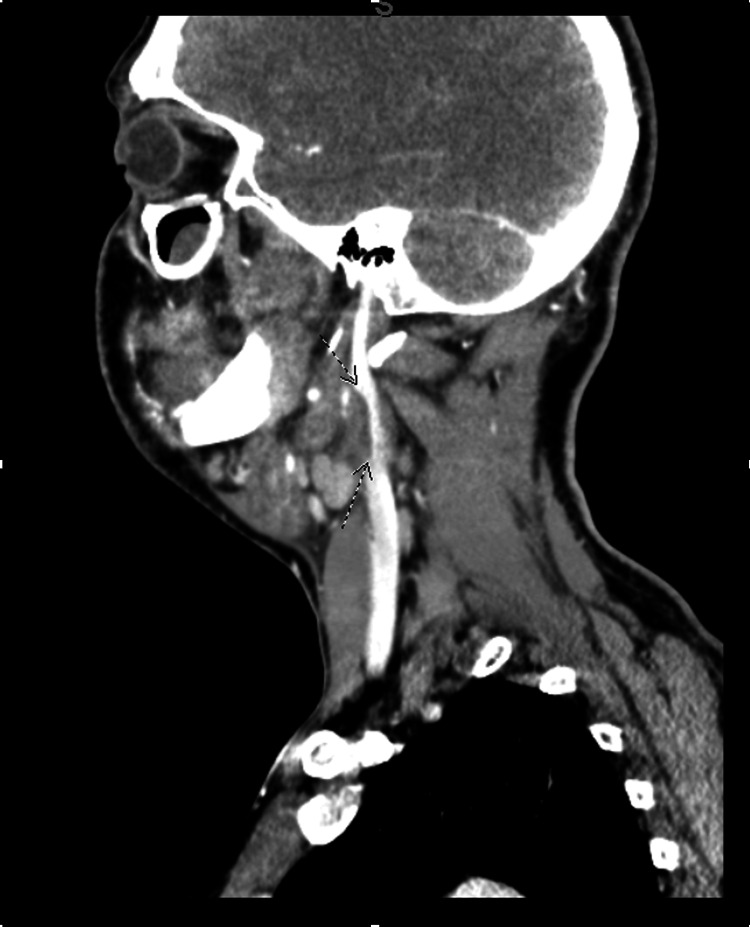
Initial sagittal contrast-enhanced CT scan demonstrating partial occlusion of the left internal jugular vein (IJV), as indicated by the arrows. The IJV with contrast filing defects suggests early signs of thrombophlebitis.
